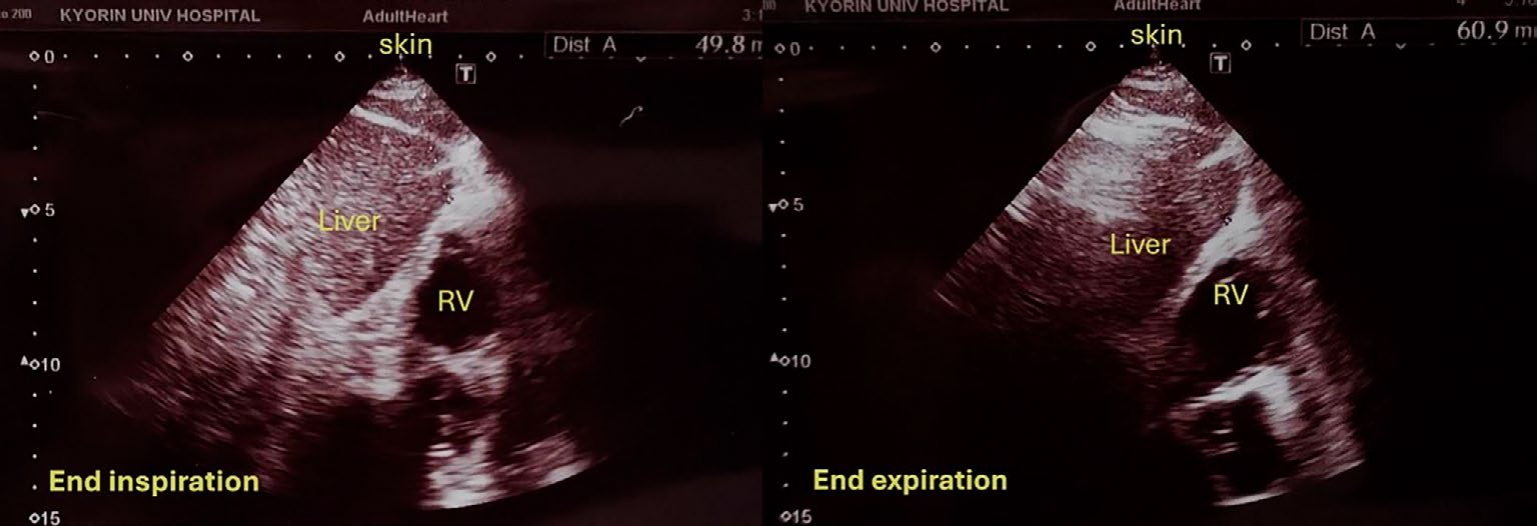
Ultrasound image demonstrating a representative case in which the liver overlaps the subxiphoid trajectory to the epicardial space during both inspiratory and expiratory phases, with more pronounced overlap during expiration. The image was obtained using the anterior (midline‐directed) probe orientation. RV, right ventricle.
